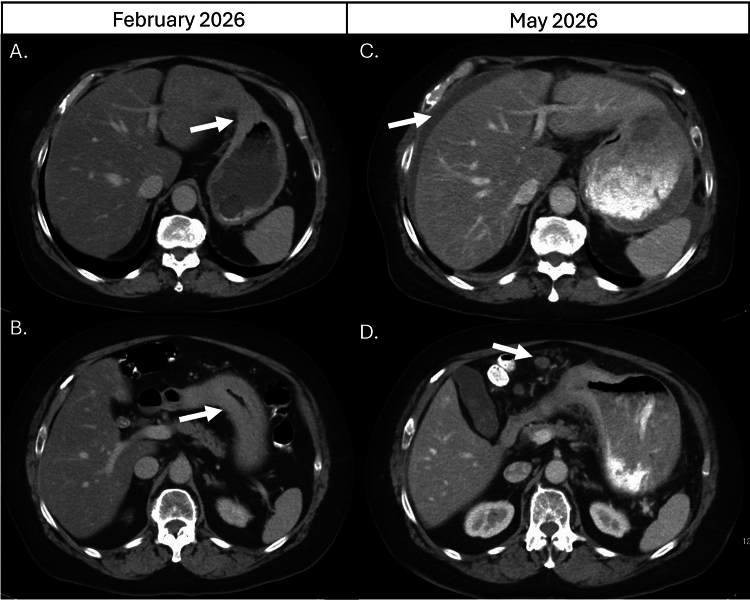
Images from a 68 year-old patient who presented with early satiety and belching (dyspepsia) and was first evaluated with a computed tomography in a rural setting. A, B A scan from February 2026 showed distal gastric wall-thickening without adenopathy or other findings. The same patient had persistent symptoms after proton pump inhibitor therapy, and a repeat scan exactly 3 months later in May 2026 demonstrated C interval development of malignant ascites and D bulky peri-gastric adenopathy
